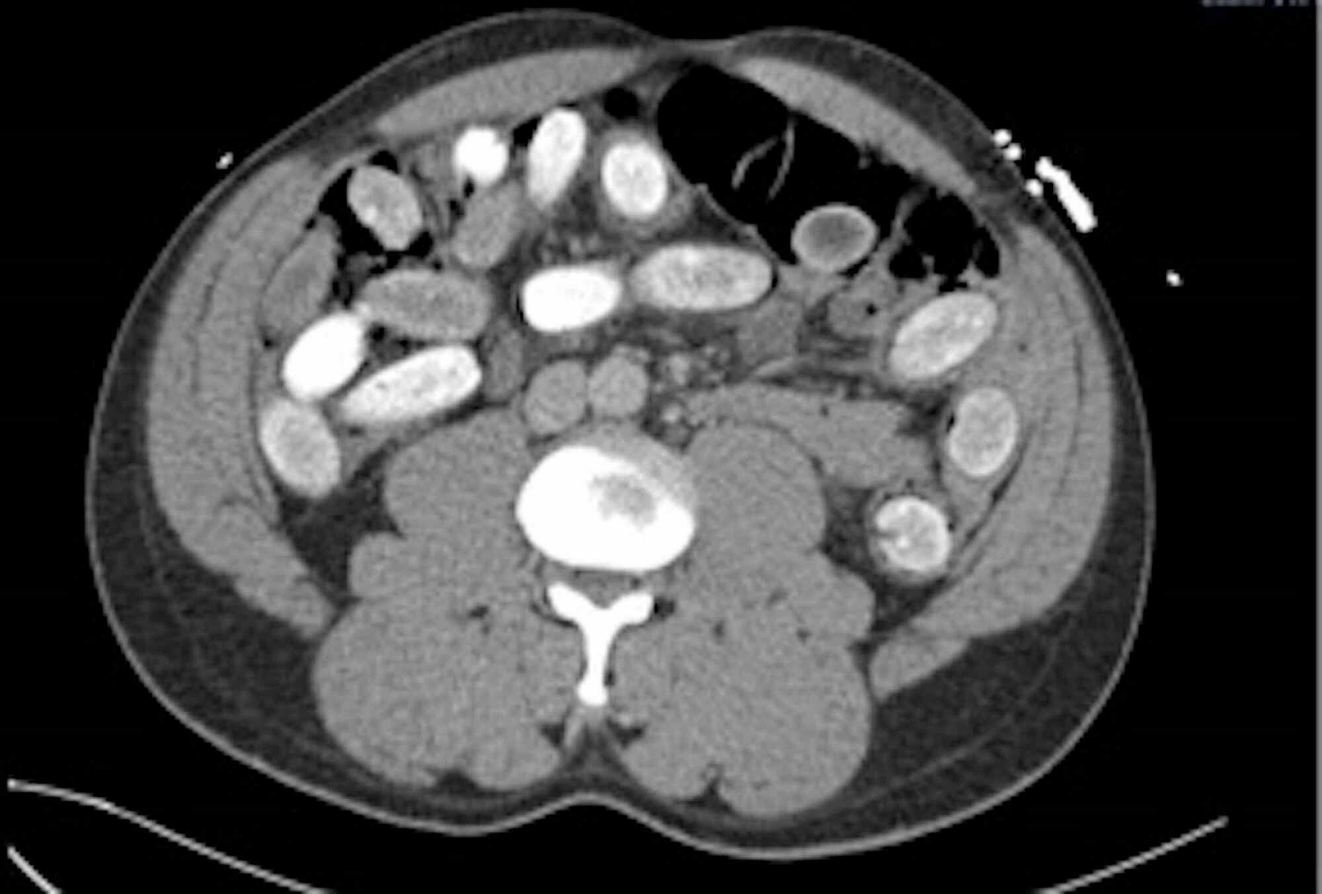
Axial view of a CT scan showing the cocaine packets distributed in the abdomen.
